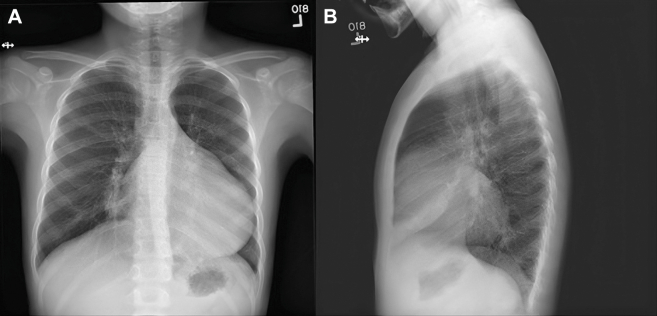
Chest X-ray Chest x-ray with coronal plane (A) and sagittal plane (B), revealing an enlarged cardiomediastinal silhouette and contours, which suggest a mediastinal abnormality.
